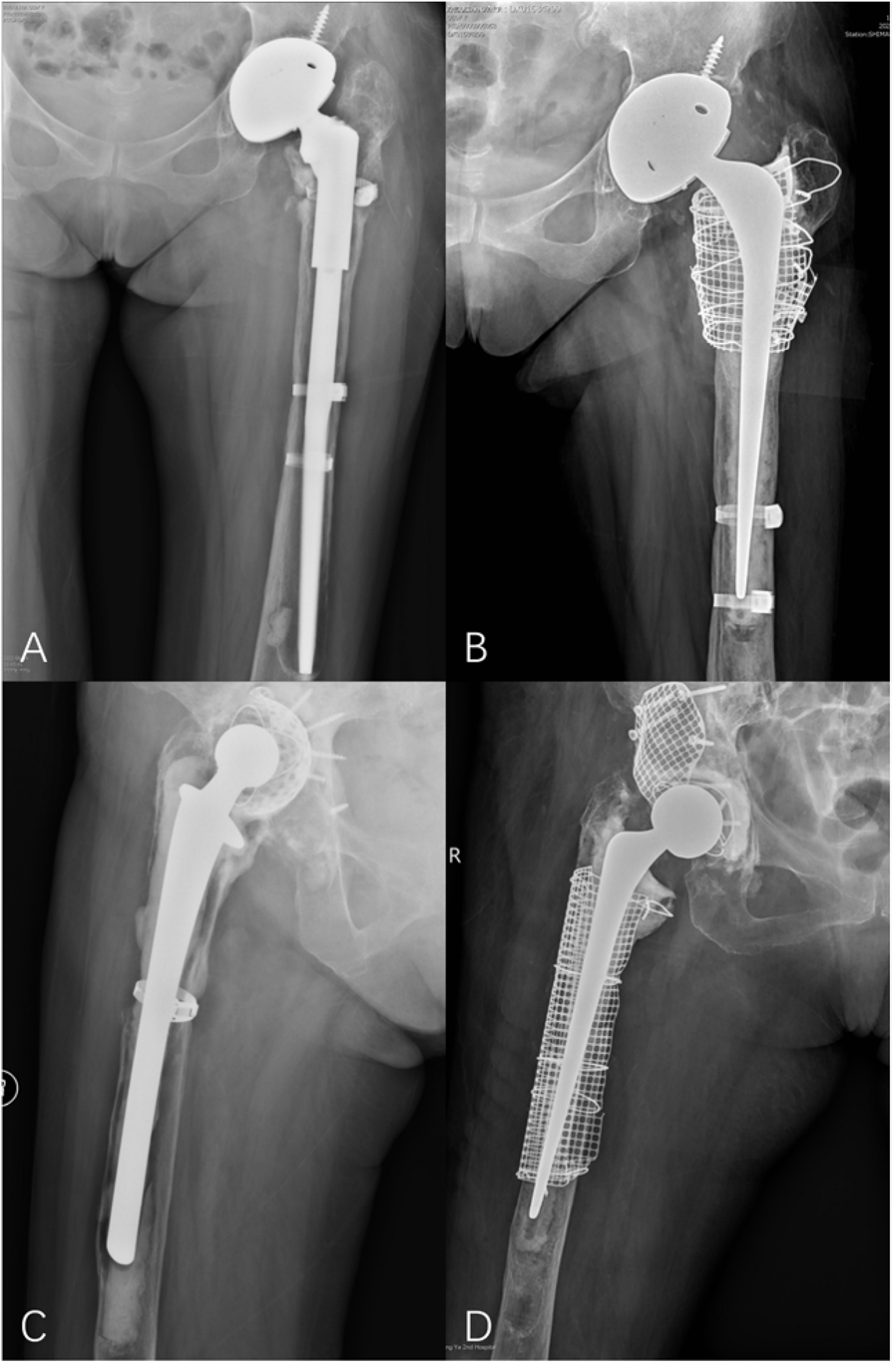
A, B Pre-op and Post-op X-ray of one case with Paprosky Type III defect; C, D Pre-op and Post-op X-ray of one case with bilateral Paprosky Type IV defect
